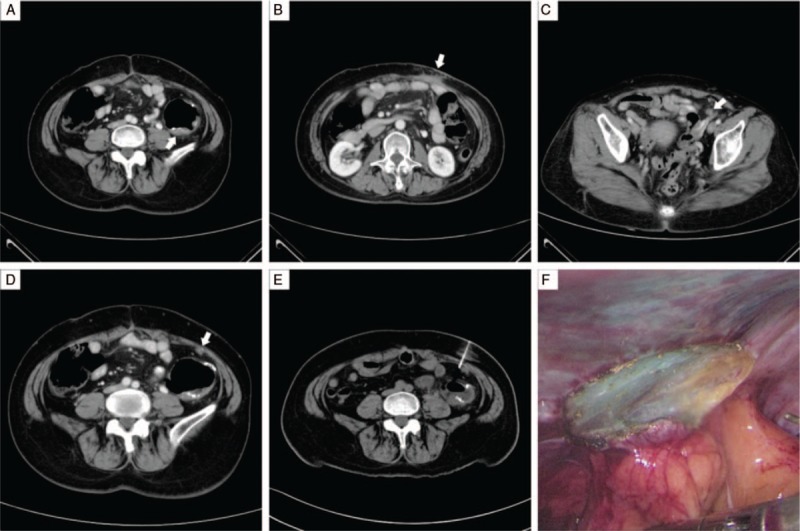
(A) Preoperative CT scan in Case 2 showing local recurrence at the anastomotic site (arrow). (B–D) Three sites of tumor implants involving the anterior abdominal wall and left lower peritoneum (arrows). (E) CT-guided needle localization of the target nodule in the left lower abdomen. (F) Endoscopic image showing successful blue coloration of the target nodule. CT = computerized tomography.
